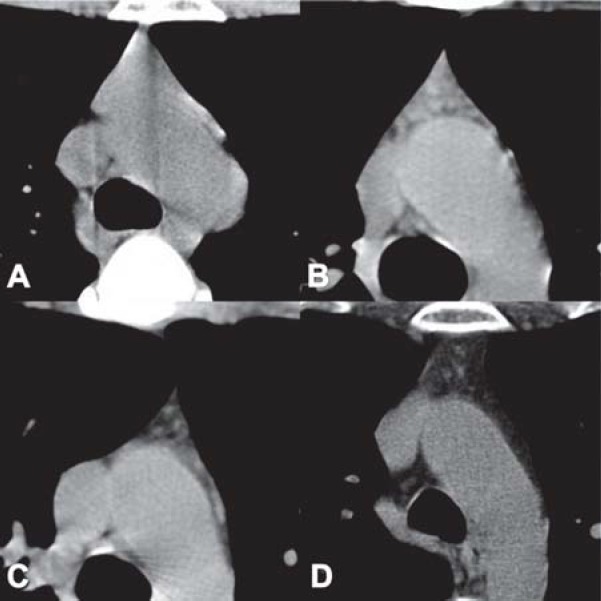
Progressive fatty infiltration of the thymus. (A) 20-y/o female with myasthenia gravis; CT scan shows remaining thymic mass with density similar to muscle; pathologic diagnosis - thymic hyperplasia. (B) 28-y/o female, with myasthenia gravis; CT scan shows remaining thymic mass with early fatty infiltration; pathologic diagnosis – thymic hyperplasia. (C) 32-y/o female with myasthenia gravis; CT scan shows remnant of thymic tissue; pathologic diagnosis - thymic atrophy. (D) 46-y/o female with myasthenia gravis; CT scan shows replacement of the thymus by fat; pathologic diagnosis - thymic atrophy [4].
